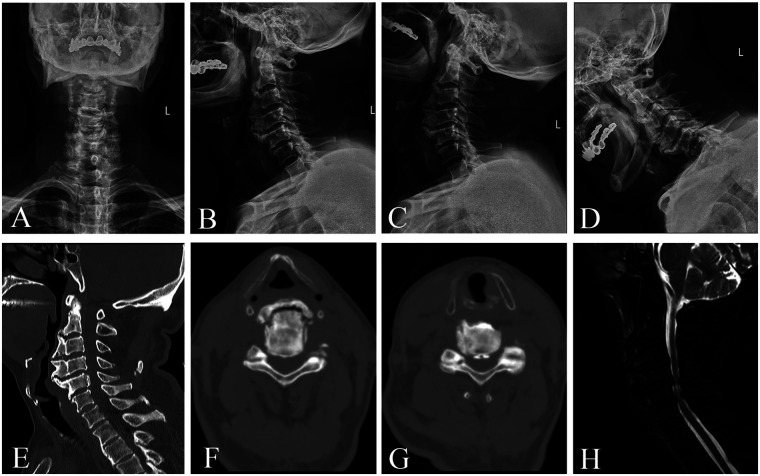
(A,B) cervical spine radiographs, AP and lateral views. (C,D) Cervical Flexion-Extension Radiographs. (E–G) Sagittal reformatted and axial CT images of the cervical spine at the C4 and C5 levels. (H) Sagittal T2-weighted magnetic resonance imaging (MRI) of the cervical spine.
